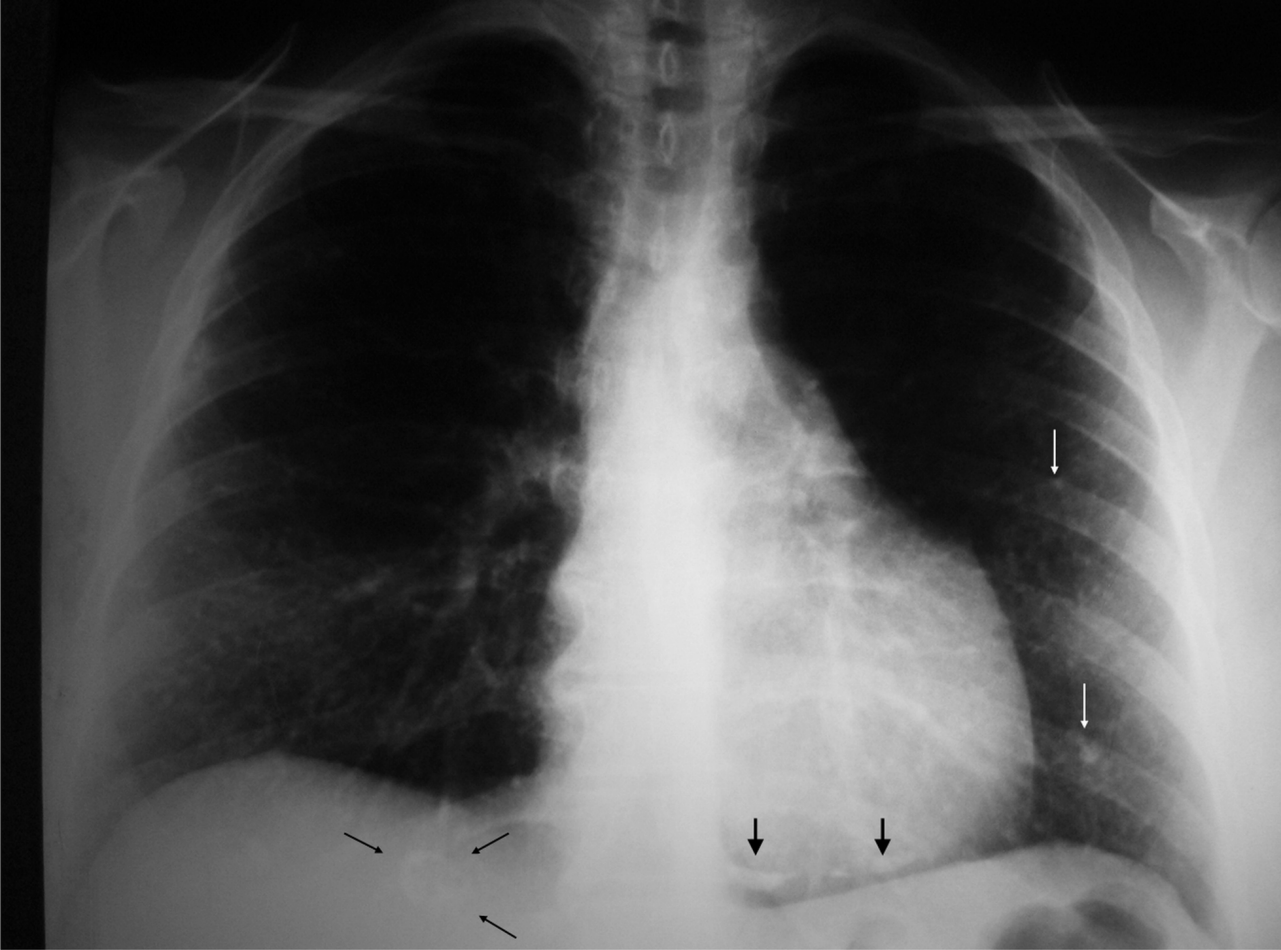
Chest X-ray. Tiny calcified nodules are visible on lung fields (white arrows). A coarse calcification of the heart cyst is projected at the heart base (thick black arrows). A calcified mass is also identified at the liver (thin black arrows).
